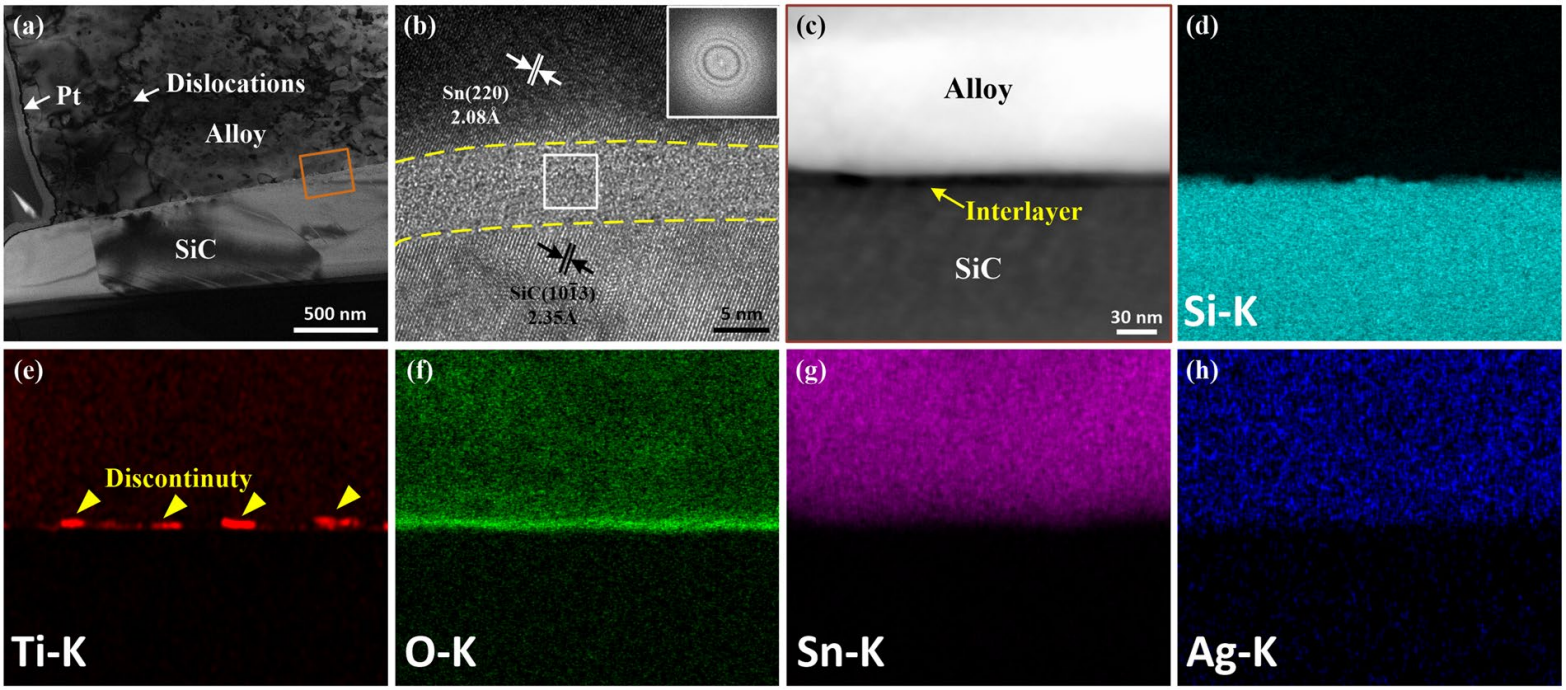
(a) Bright-field cross-sectional TEM image of the interface soldered with ultrasonic action for 0.1 s; (b) high-resolution TEM (HRTEM) image of the interface, and the inset was obtained by fast Fourier transform (FFT) of the white rectangular area; (c) HAADF image of the orange range in (a) and the corresponding STEM-EDS maps of (d) Si, (e) Ti, (f) O, (g) Sn, and (h) Ag.
